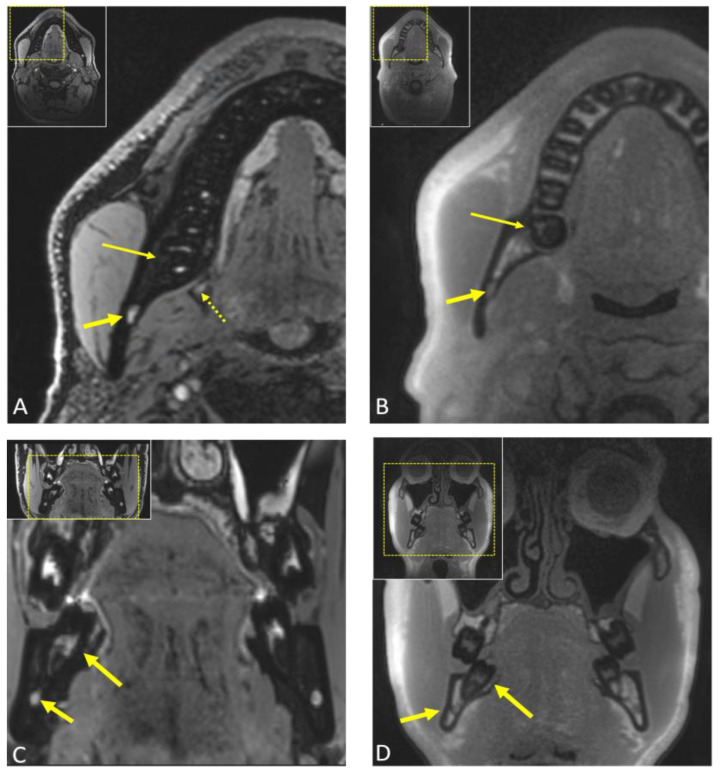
(A) Axial and (C) coronal reconstruction of a preoperative 3D double-echo steady-state (DESS) MRI of a patient undergoing third molar surgery [56]. In (A), the long arrow indicates the mandibular third molar, while the short arrow points to the entry of the inferior alveolar nerve, depicted as a hyperintense signal in the mandibular canal. The dotted arrow points to the lingual nerve at the level of the third molar. In (C), the long arrow points to the tooth 48, and the short arrow visualizes the precise localization of the inferior alveolar nerve within the osseous boundaries of the mandibular canal. (B) Axial and (D) coronal images display reconstructions from a preoperative 3D ultrashort echo time (UTE) MRI, which is an MRI sequence known for producing CT-like MRI images, particularly suitable for imaging osseous tissue. In (B,D), the long arrow points to the mandibular third molar, while the short arrow points to the inferior alveolar nerve.
